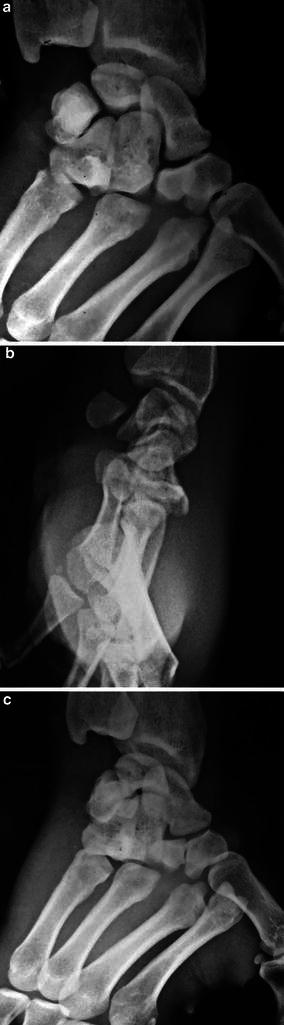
Postinjury radiographs (anteroposterior, lateral, and oblique views) showing volar and radial dislocation of the second, third, and fourth carpometacarpal joints
